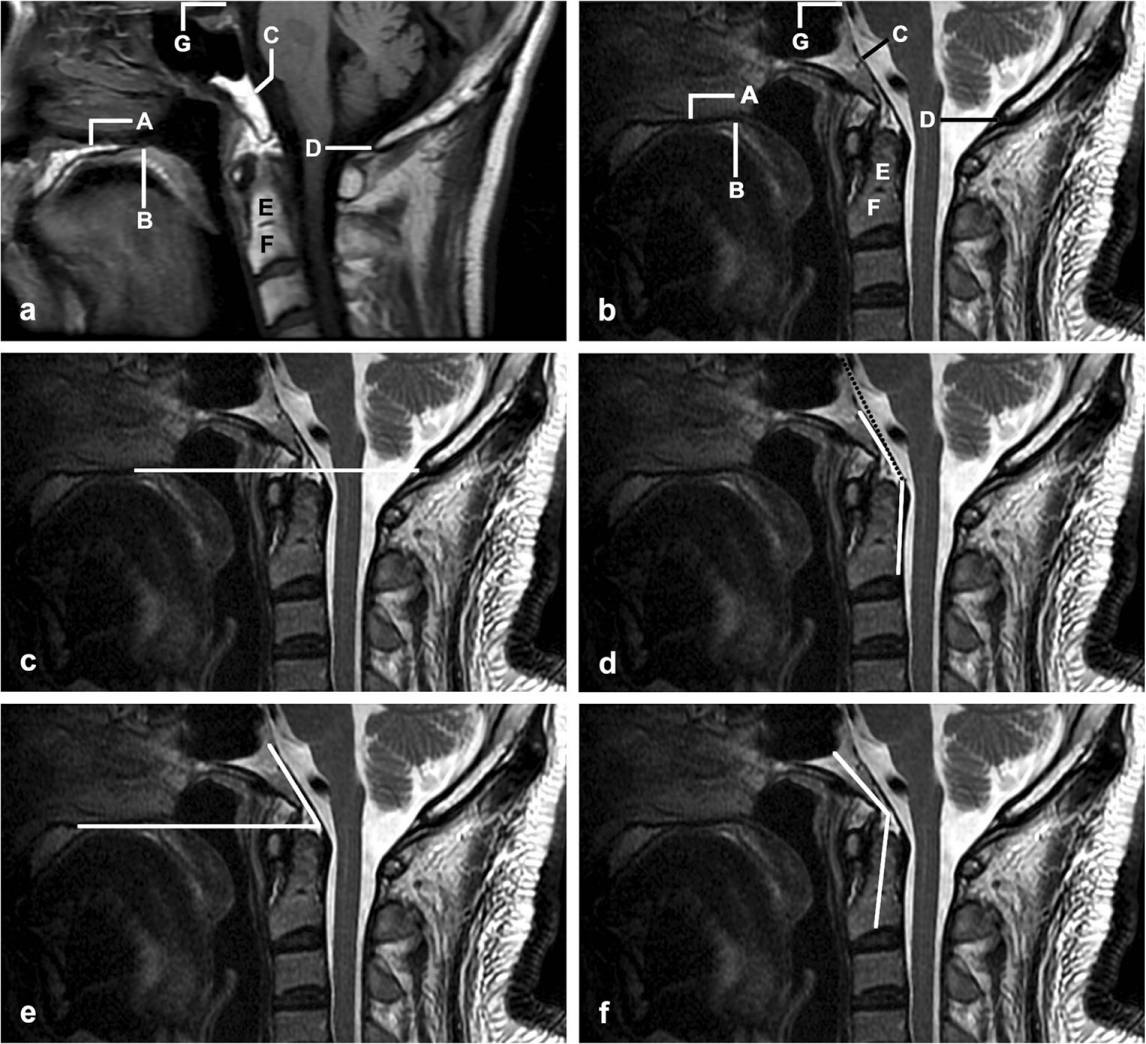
Lines and angles for the evaluation of basilar invagination. a, b Normal landmarks on midsagittal MRI T1-weighted and T2-weighted images, respectively. (A) Superior margin of the hard palate plane. (B) Dorsal margin of the hard palate. (C) Clivus. (D) Opisthion (dorsal margin of the foramen magnum). (E) Odontoid process. (F) Body of the axis. (G) Dorsum sellae. c Chamberlain’s line, the dorsal margin of the hard palate to the opisthion. d Clivoaxial angle (CXA), the angle formed at the junction of the newer Wackenheim line (white line, from the spheno-occipital synchondrosis to the top of the odontoid process) and the line along the posterior surface of the axis body and odontoid process. The dotted black line represents the traditional Wackenheim line from the top of the dorsum sellae to the top of the odontoid process. e Clivopalate angle (CPA), the angle formed at the junction of Wackenheim line and the hard palate line. f Clivodens angle (CDA), the angle formed at the intersection of a line along the long axis of the clivus and the one along the long axis of the odontoid process
